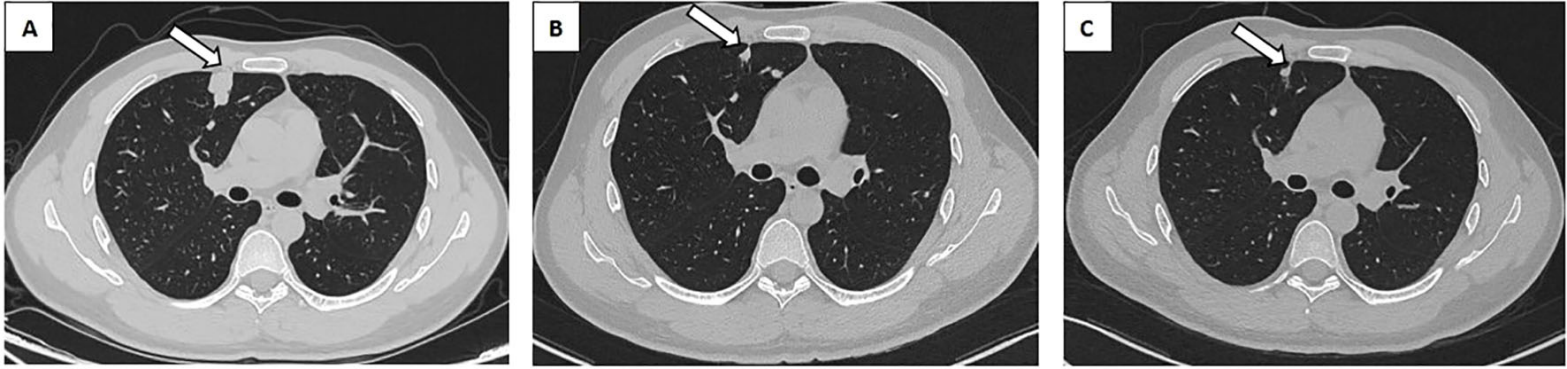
Chest CT revealed a metastatic tumor in the right lung. (A) Metastatic tumor of the right lung prior to chemotherapy (white arrow). (B) The BEP regime for two cycles of chemotherapy for right lung metastasis (white arrow). (C) The TIP regime for one cycle of chemotherapy for right lung metastasis (white arrow). CT, Computed Tomography; BEP, Bleomycin, Etoposide, Cisplatin; TIP, Paclitaxel, Ifosfamide, Cisplatin.
